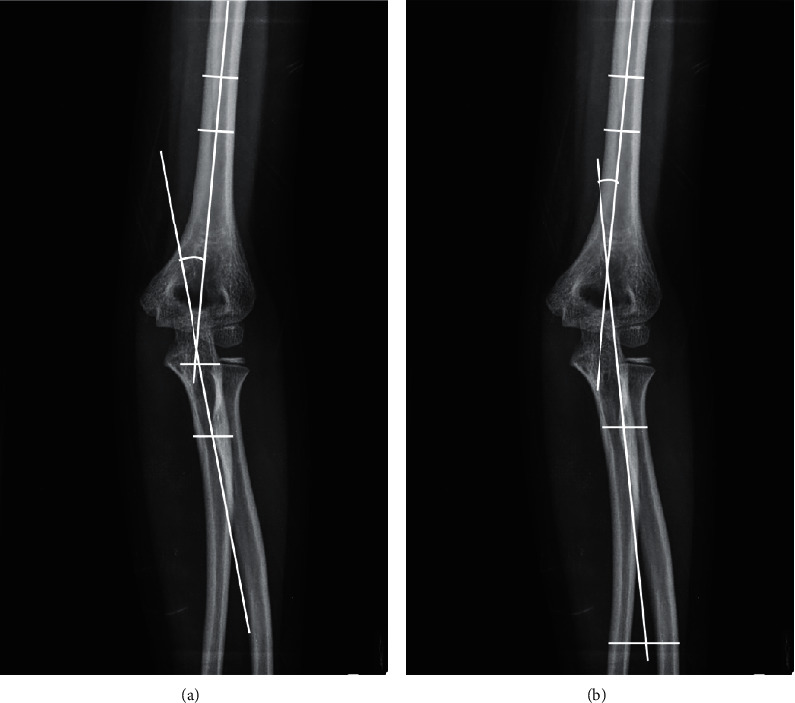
Radiographic measurements. The carrying angle (a) is the angle between the longitudinal axis of the humeral shaft and the longitudinal axis of the shaft of the ulna. The humerus-elbow-wrist angle (b) is the angle between the longitudinal axis of the humeral shaft and a line passing through the midpoints of 2 transverse lines across the forearm.
